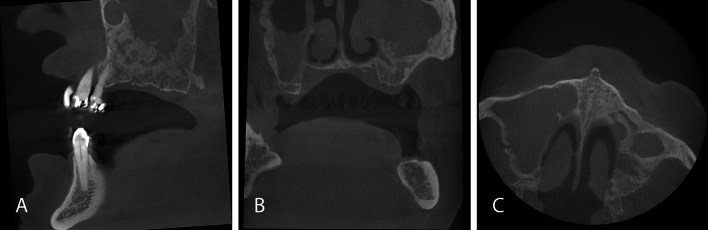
CBCT scan for digital implant planning with large FOV (10 × 10 cm) in a partially edentulous 79-year-old male patient. Opacification (over 50%) of the partially imaged left maxillary sinus antrum with inhomogeneous osseous appositions basally and absence of the left lateral nasal wall is conspicuous. In the right maxillary sinus, an opacification was present in the lower half of the antrum. Due to the incidental findings in the maxillary sinuses, further evaluation by an otorhinolaryngologist was indicated. Sagittal (A), coronal (B) and axial (C) images
